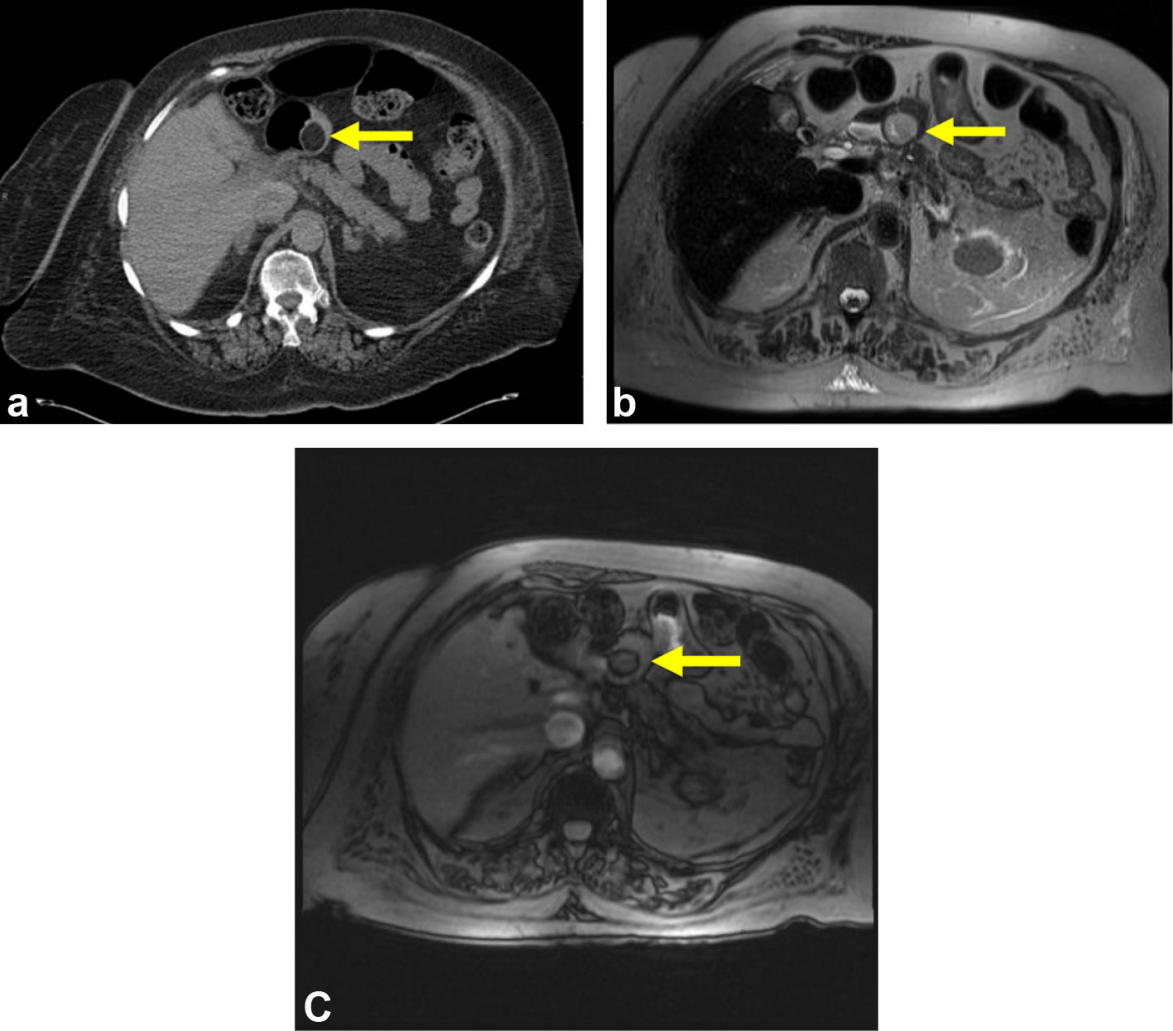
CT and MRI images of a single gastric lipoma. (a) Axial non-contrast CT of the abdomen
